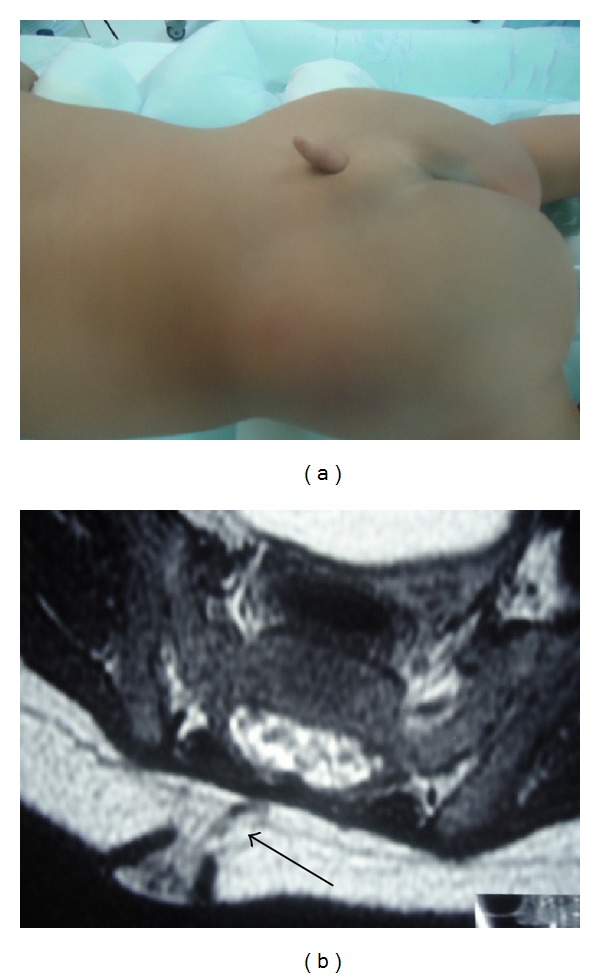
(a) An appendage lesion with approximately 2 cm with no communication with the spinal cord. (b) Confirmation by magnetic resonance image (axial image), with development of a false human tail.
